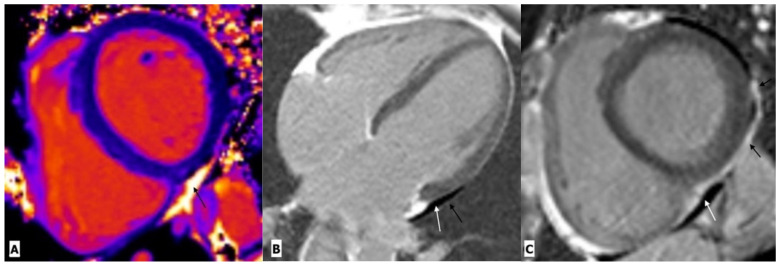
Cardiac magnetic resonance imaging in a 38-year-old man with HIV-related pericarditis and pericardial effusion. (A) Mid-ventricular short-axis view T1 map showing high signal intensity pericardial effusion (black arrow). (B) Four chambers view and (C) mid-ventricular short-axis view gadolinium enhancement images showing mild pericardial effusion (white arrow) with thickening and enhancement of pericardium (black arrow) along the left lateral LV wall.
